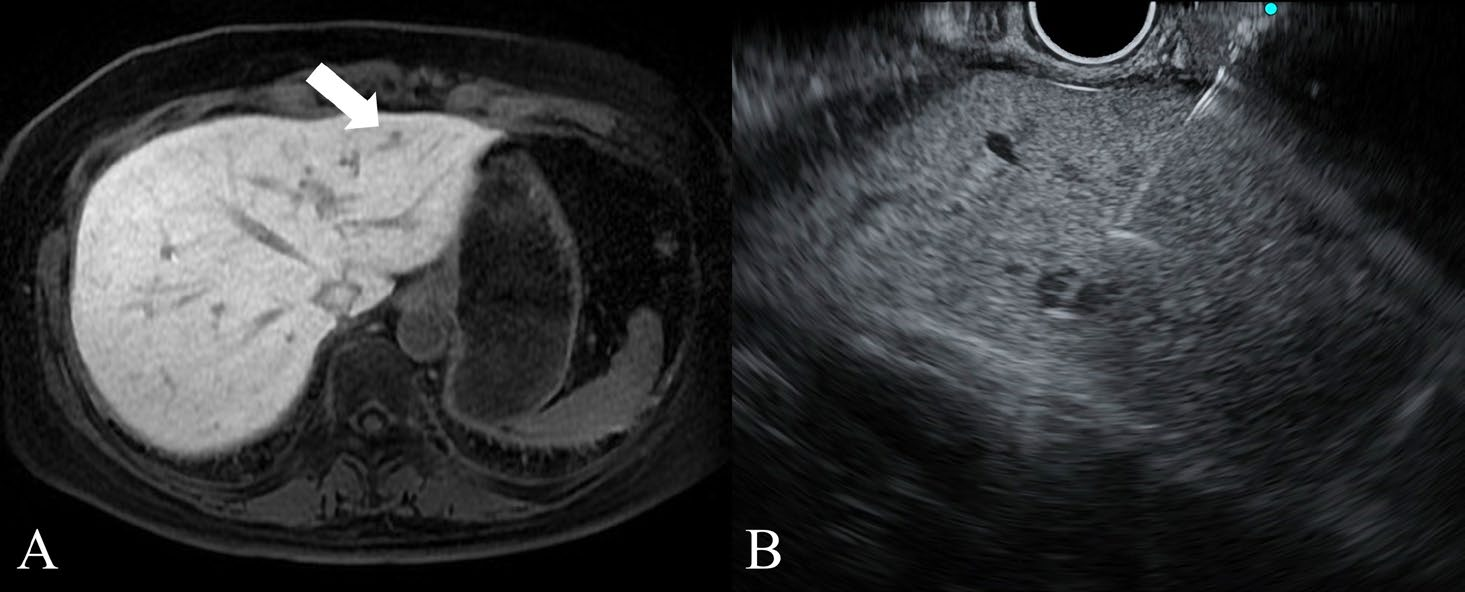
(A) Contrast‐enhanced magnetic resonance imaging revealed a small focal liver lesion (< 10 mm), located in segment 3 of the liver's lateral section (arrow). (B) EUS‐TA was performed to obtain a sample from the focal liver lesion.
